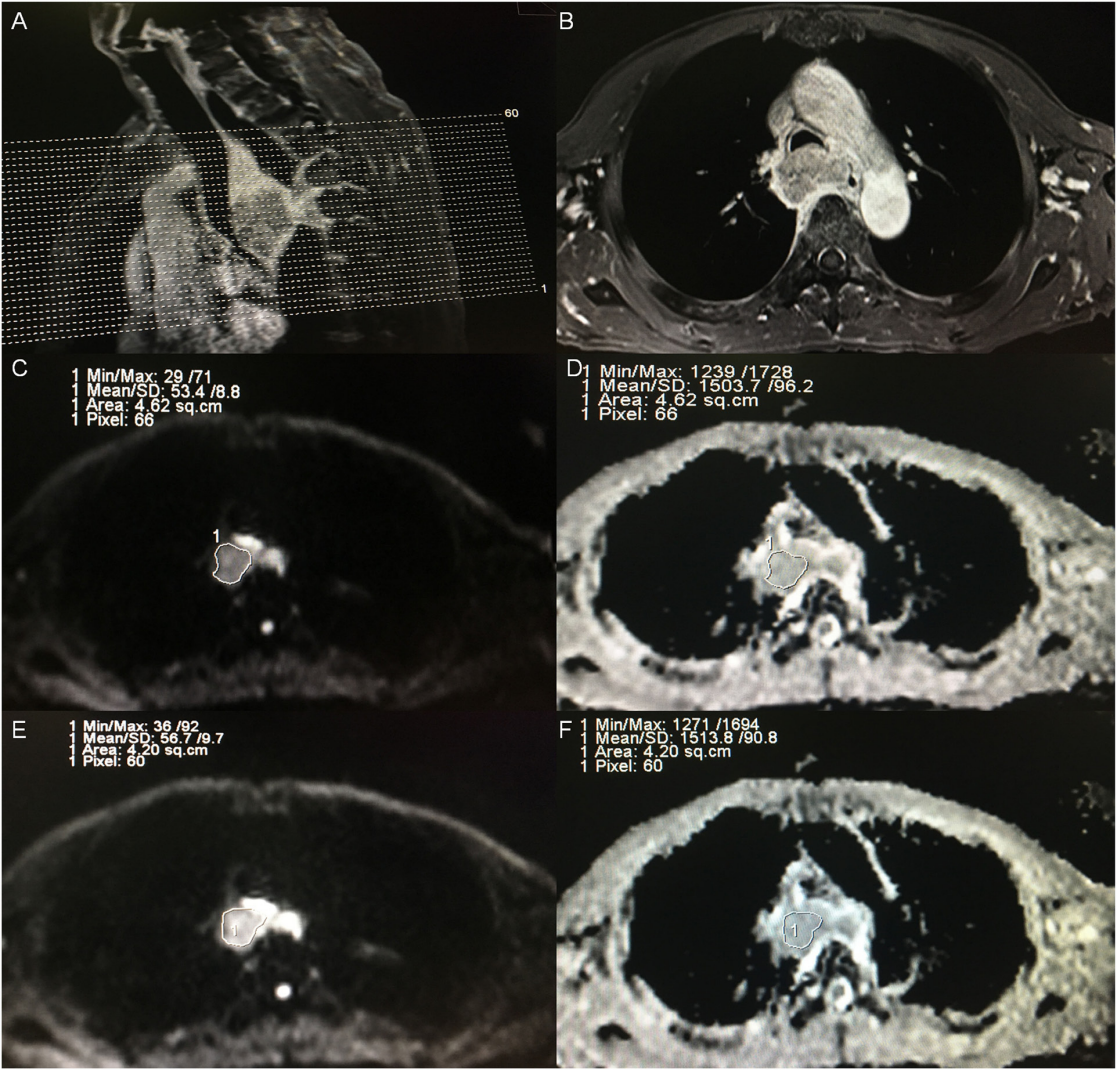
Example of ADC measurements of a primary tumor in a patient diagnosed as esophageal carcinoma before treatment (A) A sagittal view with position line of T1-weighted plus contrast-enhanced (T1+C) image for the primary tumor. (B) A transverse view of T1+C image for the one of three continuous sections with maximal diameter of tumor according to the sagittal and transverse view. (C) A region of interest (ROI) was placed manually for observer A in the selected section, on the image obtained at a b-value of 1000 s/mm^2^, and the ROI was then copied and pasted onto the ADC map (D), and the ADC and SD of the selected section were automatically calculated. (E) A region of interest (ROI) was placed manually for observer B in the selected section, on the image obtained at a b-value of 1000 s/mm^2^, and the ROI was then copied and pasted onto the ADC map (F), and the ADC and SD were also automatically calculated.
